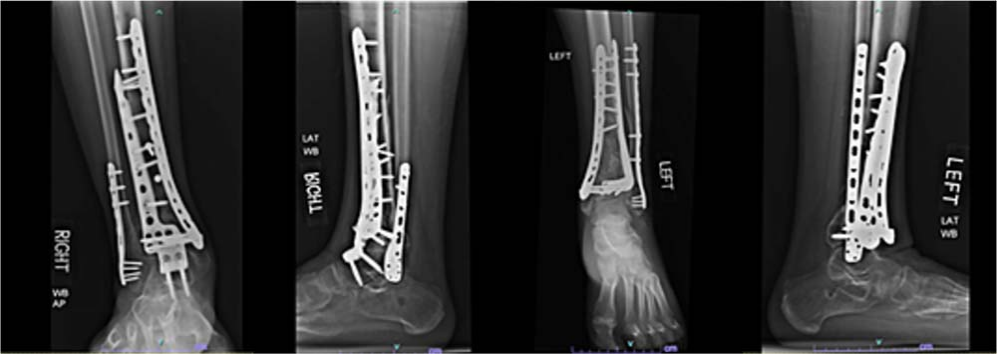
AP and lateral radiographs of patient 3 obtained 1 year and 9 months after the index procedure, demonstrating right ankle arthrodesis and a healed left ankle.
